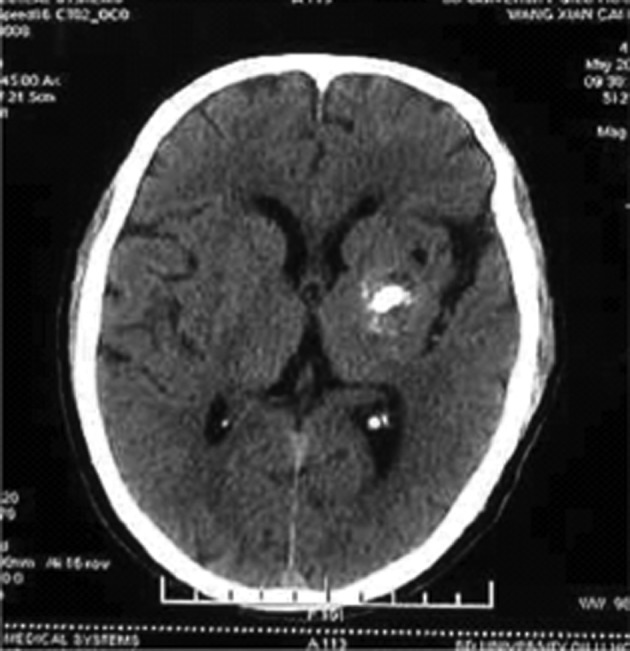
CCM on CT, the lesions complicated with hemorrhage and calcification are large enough to be visible on left basal ganglia. The foci show irregular mixed density. CCM, cerebral cavernous malformation; CT, computed tomography.
